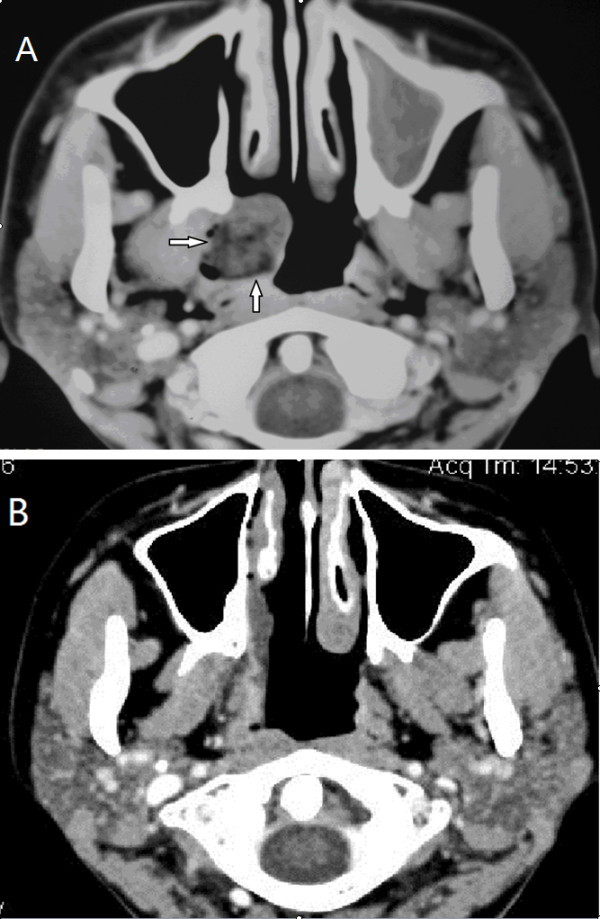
(A) Axial section of a contrast-enhanced CT scan shows the hypodense, non-enhancing lesion (arrow) in the right Eustachian tube. (B) Five months after surgery, axial section of the contrast-enhanced CT scan at the same level shows no residual tumor.
